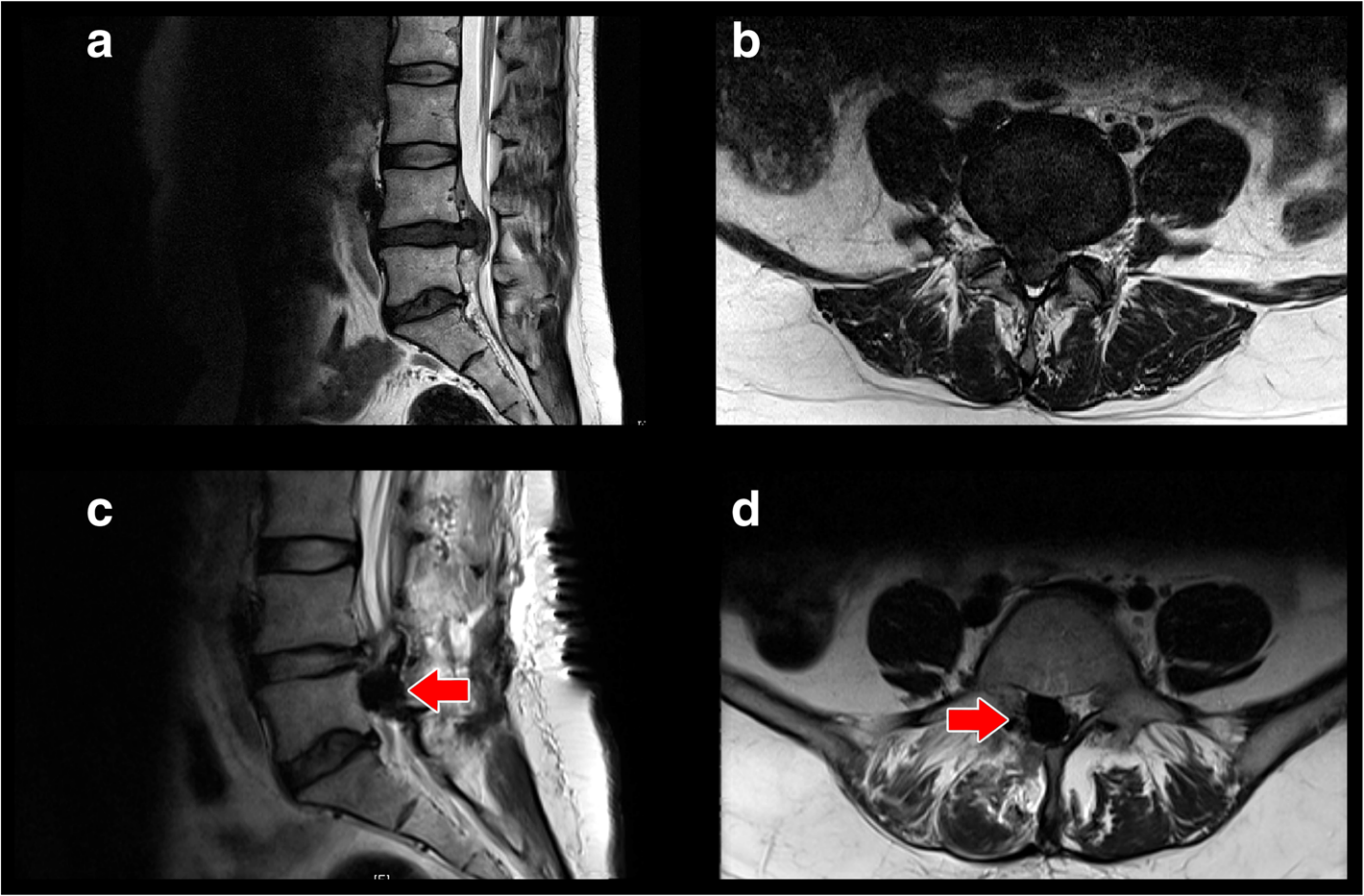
MRI T2 weight from the Lumbar spine. a and b show a massive Lumbar Disc Herniation in the Segment L4/L5 in sagital and axial views. c and d show after 36 h an early recurrent disc herniation with postoperative Bleeding (↑) and compression of the Cauda Equina causing bowel and bladder retention in sagital and axial views
